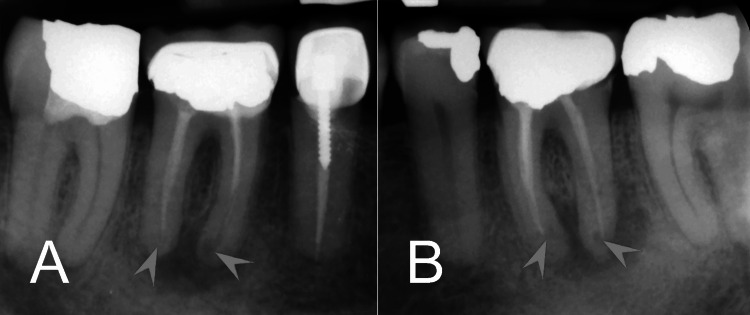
Preoperative periapical radiographs. (A) Tooth #46 showing underfilled root canals with short fillings, a large apical radiolucent lesion, and signs of IRR in the apical portions of both mesial and distal roots (arrowheads). (B) Tooth #36 displaying similar findings. IRR: internal root resorption
